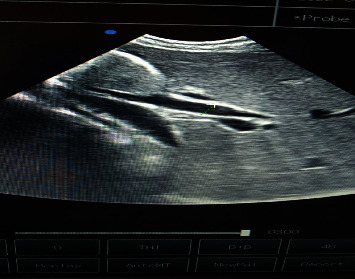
Ultrasound image showing normal common bile duct after worm exit.
